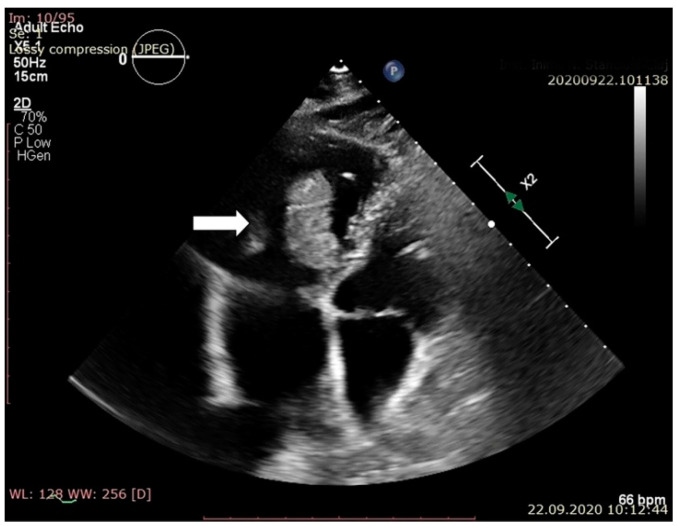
Apical 4 chambers view. Presence of the vegetation in right ventricle in diastole.
